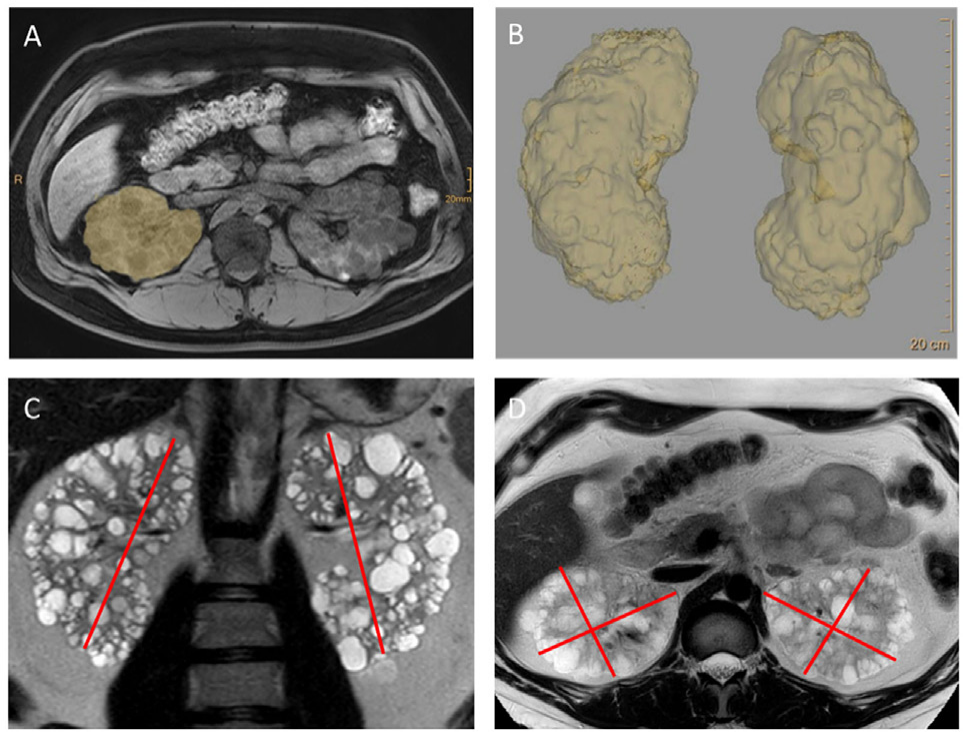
MRI imaging and volumetry of kidneys in autosomal-dominant polycystic kidney disease (ADPKD). These images reveal the classical features of ADPKD: strongly enlarged kidneys showing a ubiquitous distribution of cysts throughout the parenchyma. Kidney volume—an important prognostic feature now used in algorithm for making treatment decisions—can be obtained by planimetry (A,B) as done in the clinical trials. However, for everyday clinical decisions volumetry based on measuring the axes and using the ellipsoid formula has been shown to be sufficient (19) (C,D). From Müller et al. (27) (images kindly provided by Dr. Thorsten Persigehl, Institute of Radiology, University of Cologne).
